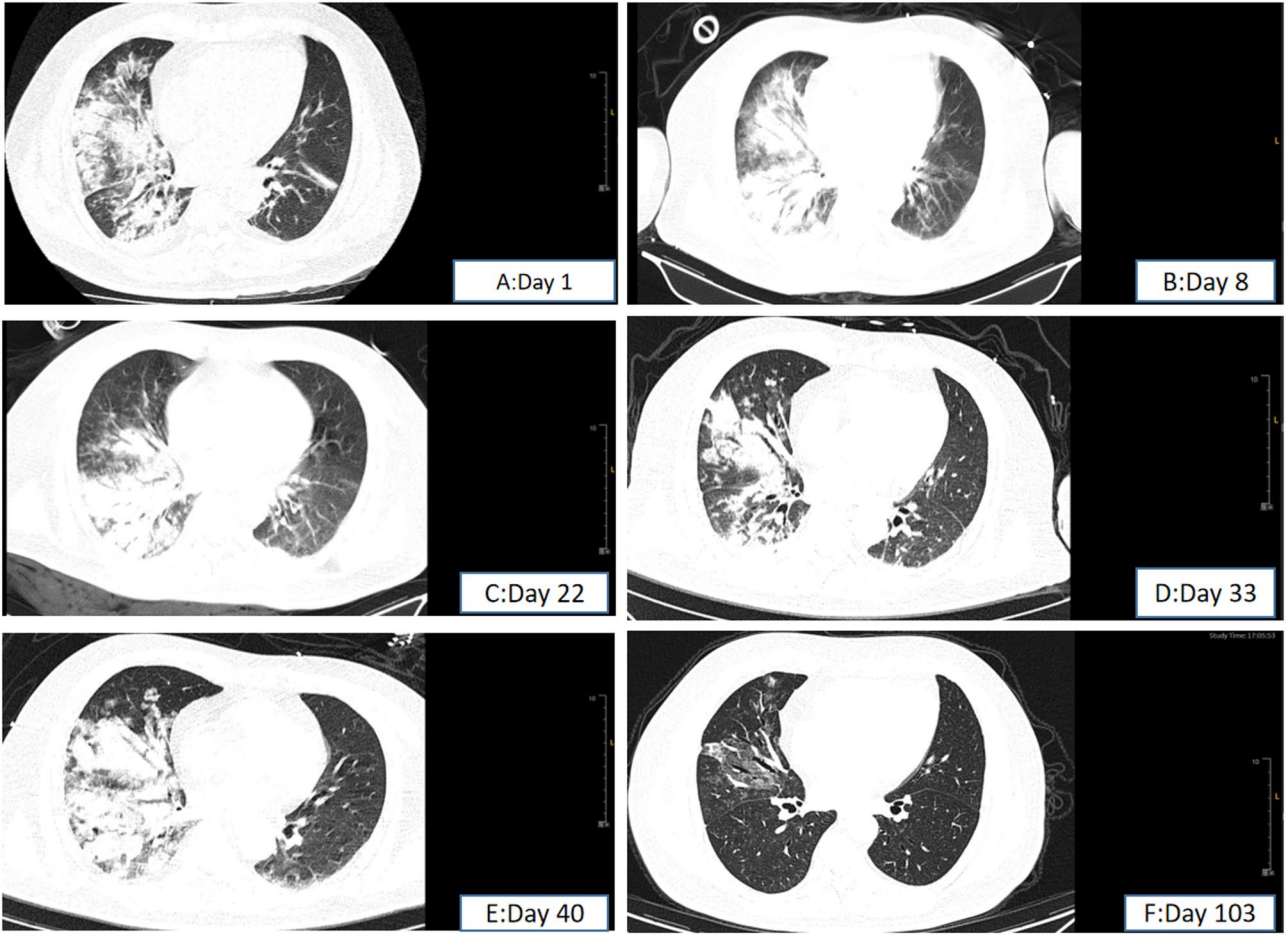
Changes in chest CT during the treatment. The day 1 is September 6th, the day of admission to Zhongshan Hospital
